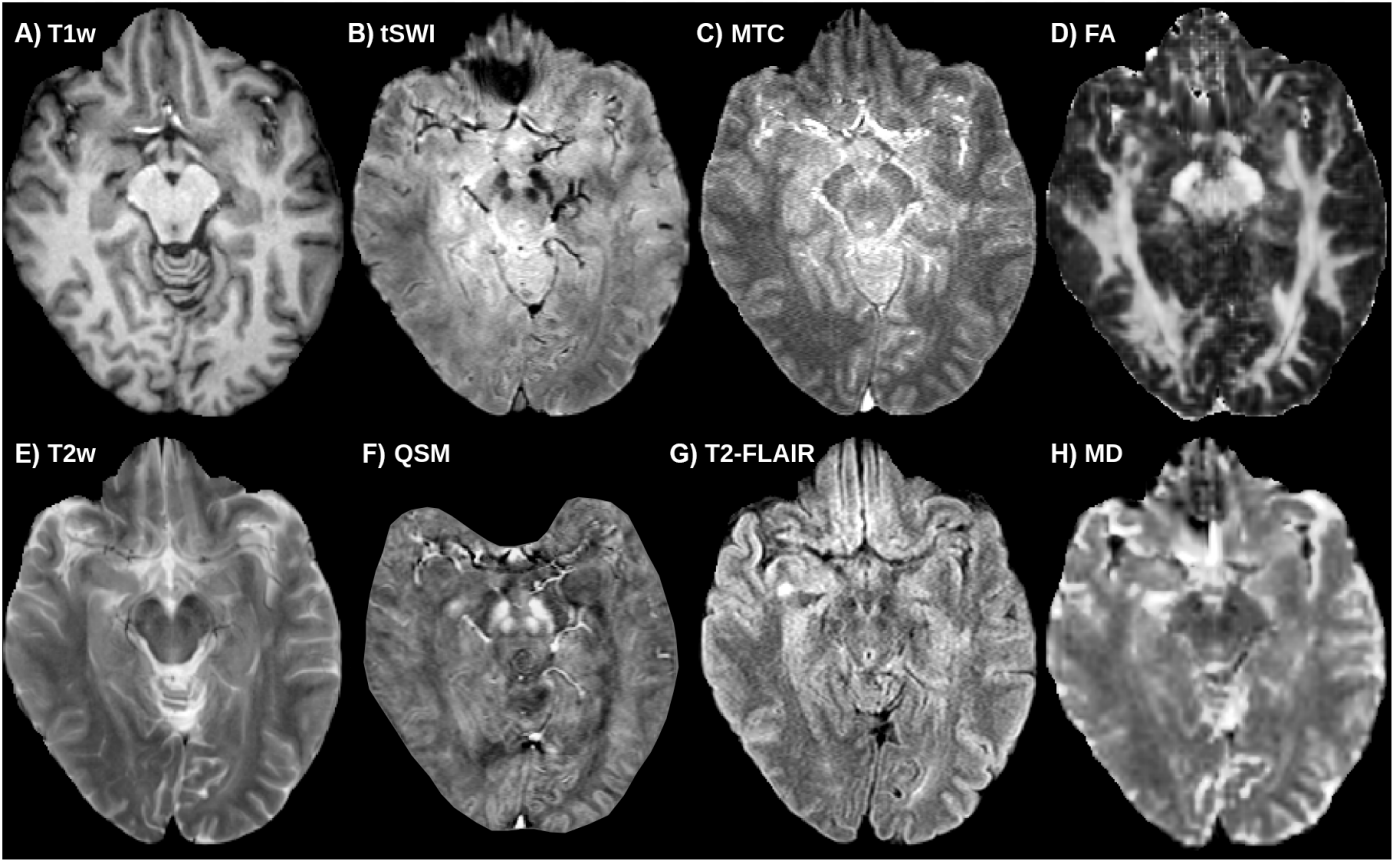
Coregistered multiparametric MRIs from a healthy control. Top row, from left: (A) T1w, (B) tSWI, (C) GRE-MTC (NM), (D) FA. Bottom row, from left: (E) T2w, (F) QSM, (G) T2-FLAIR, (H) MD.
